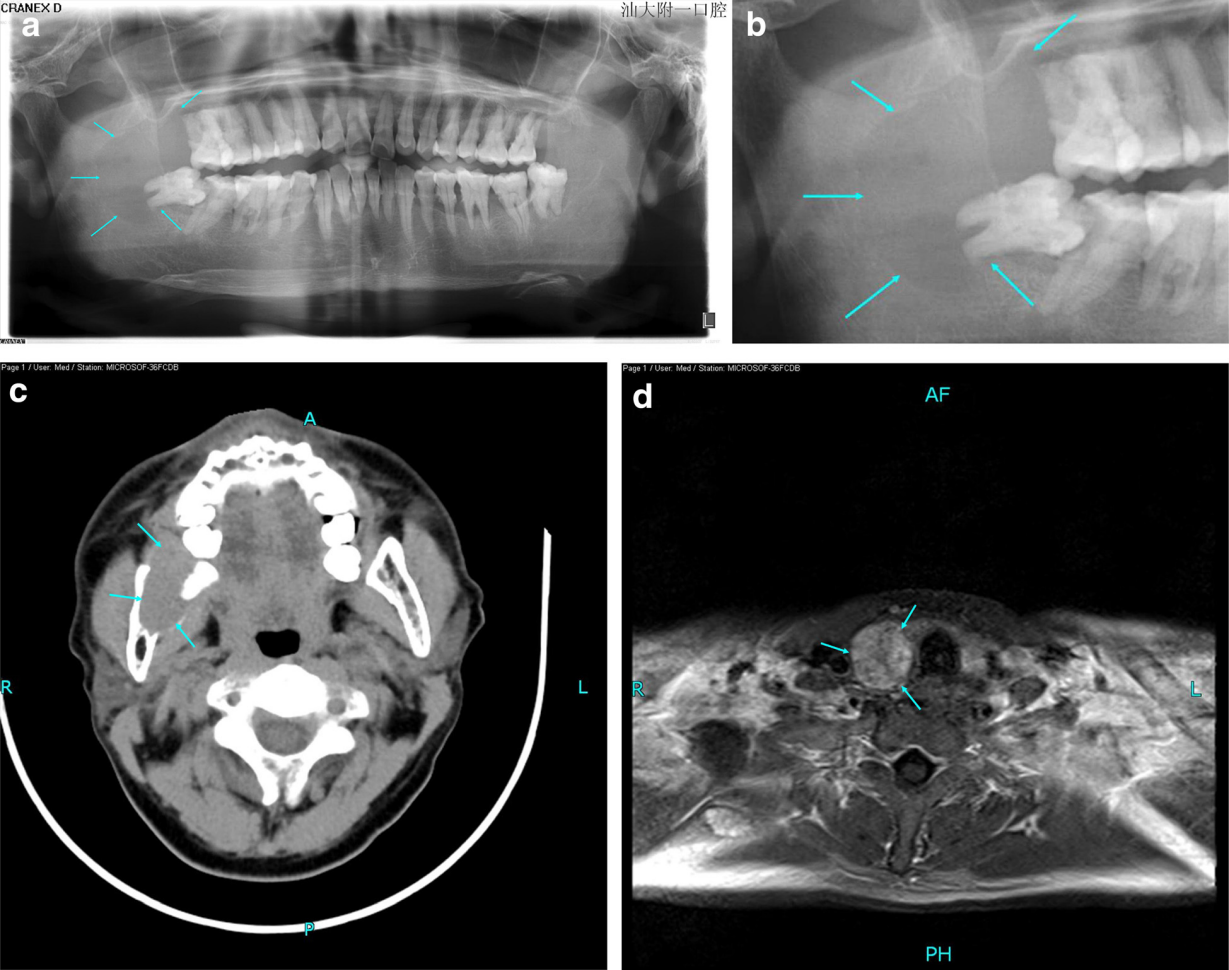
(a, b) Panoramic radiograph revealing a well-defined osteolytic lesion in the right mandible. (c) Axial CT scan showing an expansionary cystic bone lesion of the right mandible, penetrating the cortex and forming a peripheral soft tissue mass. (d) Enhanced MRI of the neck showing a 2.5-cm enhancing nodular mass on the right parathyroid
